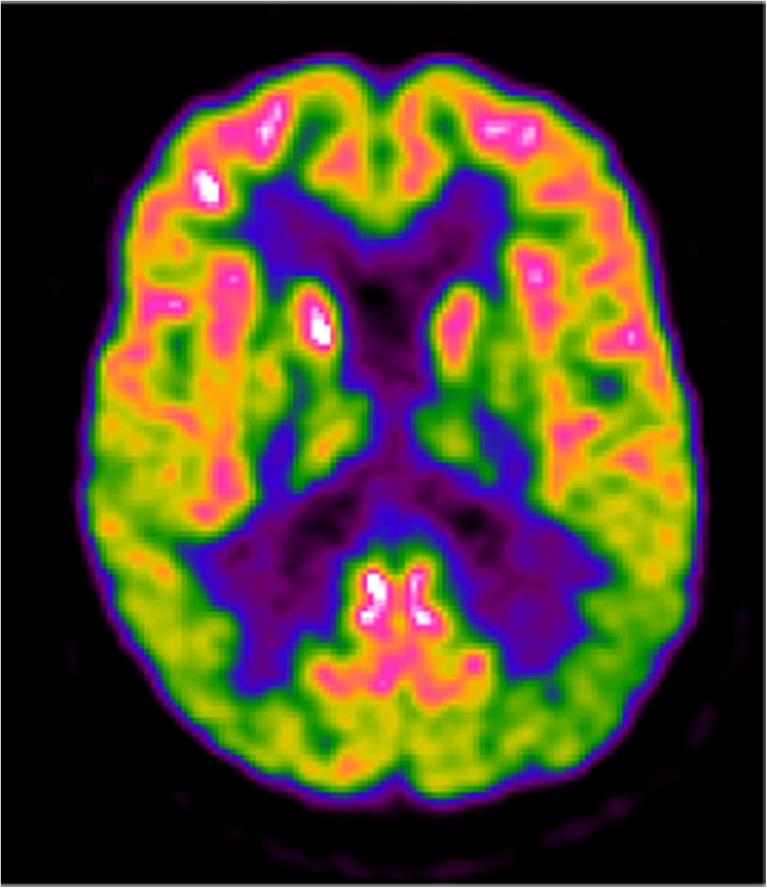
A 67-year-old patient with de novo PD and subtle cognitive impairment (amnestic MCI), MMSE score 29/30 and high educational level. The FDG PET image shows bilateral posterior parieto-occipital hypometabolism with sparing of the posterior cingulate cortex
